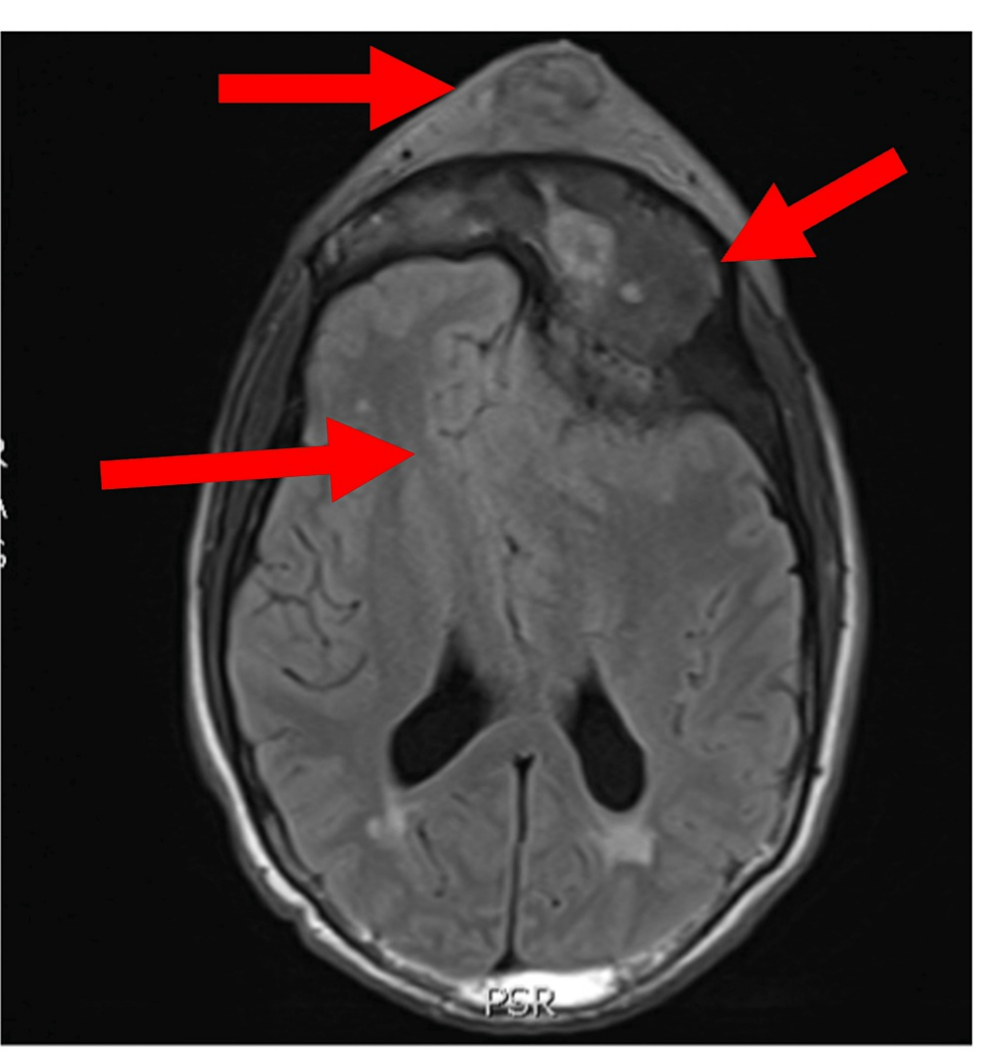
Transverse View of MRI Brain
